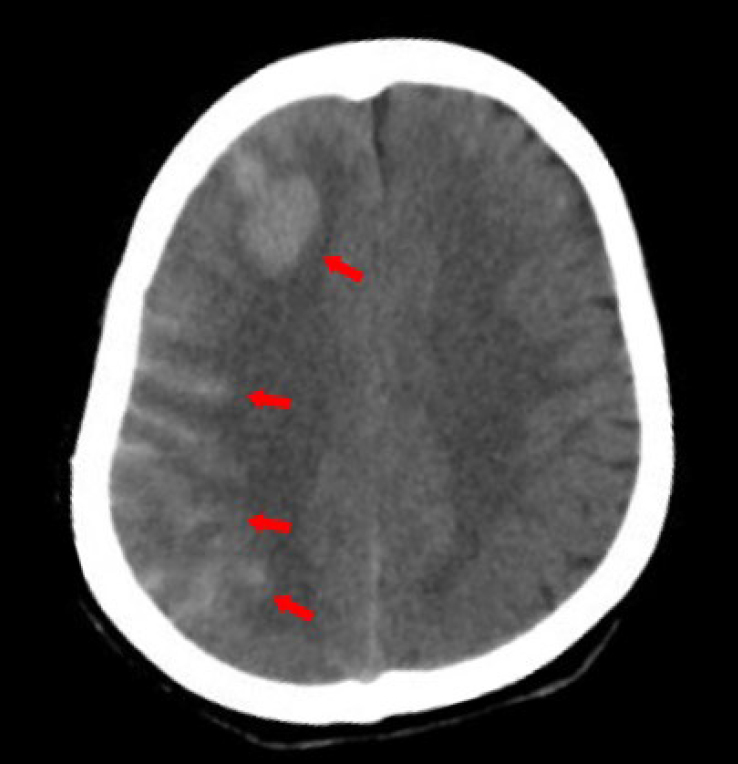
Computed tomography evidence of intracranial hemorrhage after surgery.
